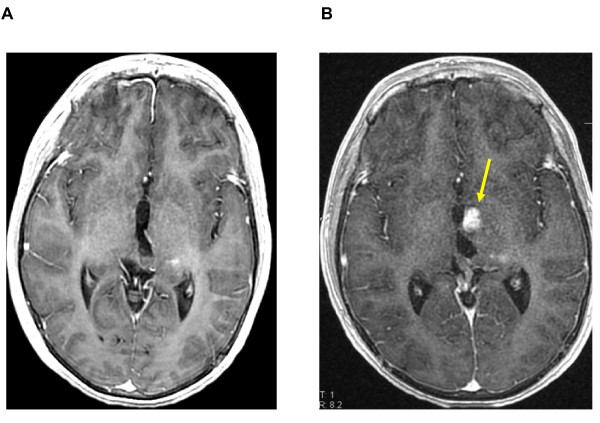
Conventional MR imaging before and six months after exclusive Proton therapy. [A] T1-weighted transaxial MR image after administration of intravenous (iv) Gd-DTPA, one week before radiation. [B] The same sequence, six months after the end of irradiation (54 Gy), showing a strong contrast enhancement with a subtle mass effect (yellow arrow).
